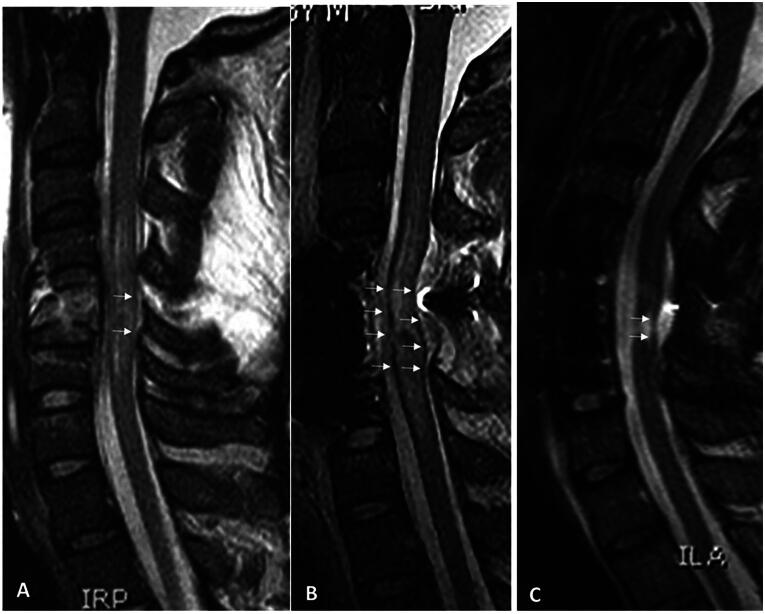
Index case tissue bridges. Midsagittal T2-weighted MRI cut indicating faint tissue bridges (arrows) in preoperative MRI (A). Clear preservation of tissue bridges (arrows) on immediate post-operative T2-weighted MRI (B). Maintained tissue bridges (arrows) 6 months following trauma in T2-weighted MRI (C) in conjunction with central syrinx.
